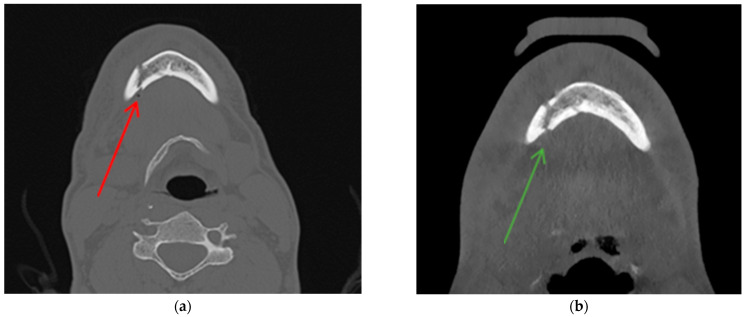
A 37-year-old patient presented with a fracture of the mandibular body on the right side and the left condylar process of the mandible. A CT scan was performed on the second day post injury (a). A CBCT scan was performed on the third day following the injury (b). The air bubble visible on the CT scan taken on the second day (red arrow) was already reabsorbed on the third day (green arrow). Therefore, we decided to reduce the number of patients and evaluate only the images taken on the second day after the injury.
